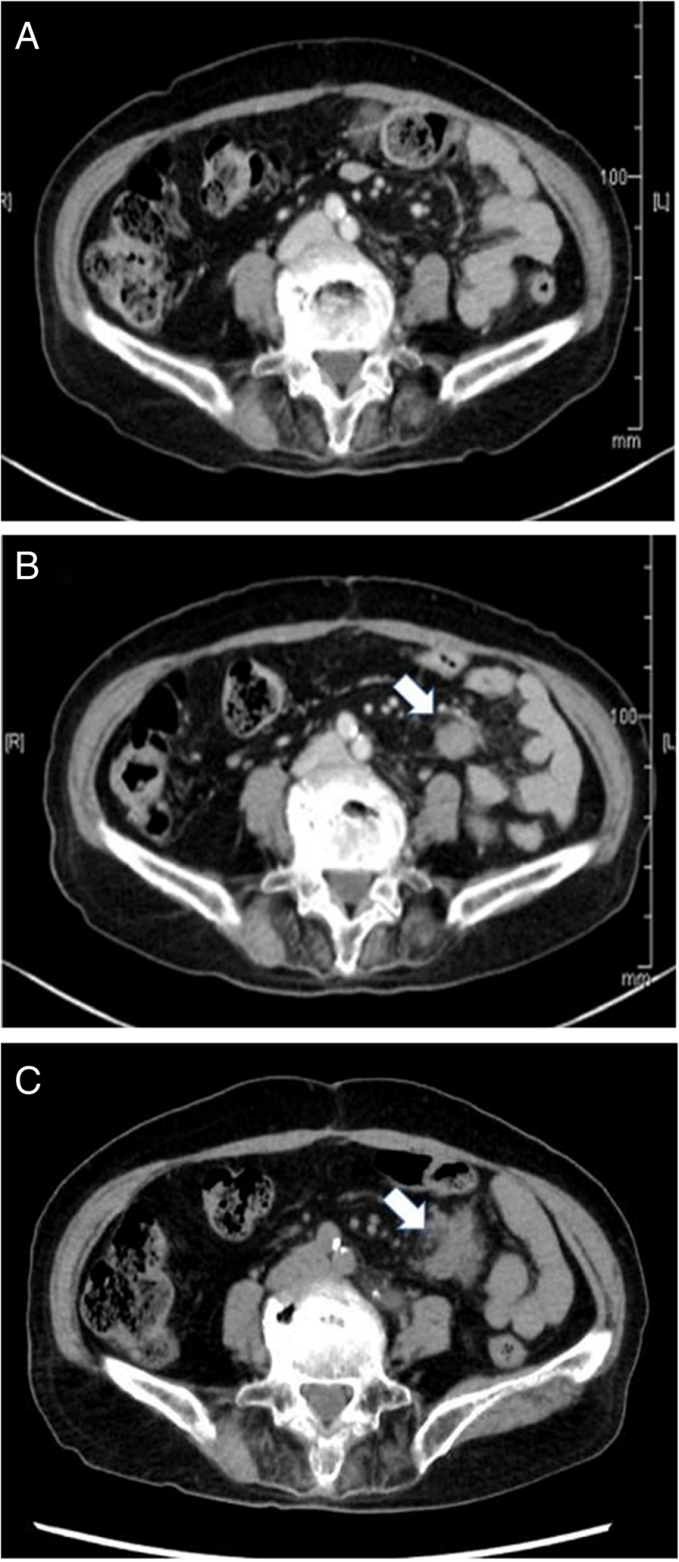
Changes in the CT findings. a No tumor is detected in the mesentery at 6 months after colectomy. b A tumor 20 mm in size was detected in the mesentery at 18 months after colectomy. CT scan showed partly unclear boundary to the surrounding tissue. c The tumor was enlarged up to the size of 33 mm with irregular margin after three courses of CAPOX + Bmab treatment
